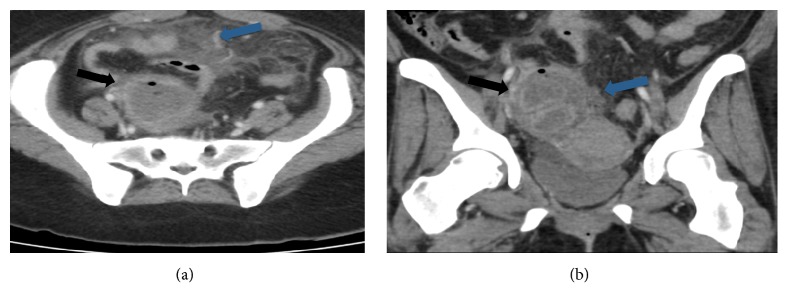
Patient's computed tomography (CT) scan of the abdomen and pelvis before surgery.  Figure 1(a) Transverse view.  Figure 1(b) Coronal view. Black arrow demonstrates right adnexal peripherally enhancing mass with extensive surrounding inflamatory changes and foci of air compatible with tubo-ovarian absess (TOA). Blue arrow demonstrates extensive inflitration of teh mesentery with inflamatory changes as well as fluid concerning for TOA rupture and seeding of the mesentary.
